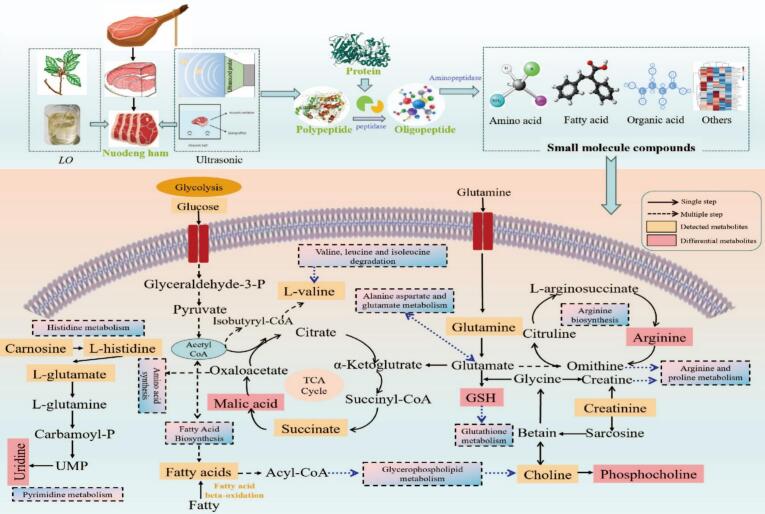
Analysis of the effect of ultrasound combined with *Litsea pungens Hemsl* essential oil compound coating on small molecule metabolic pathways network, yellow represents the metabolites identified in this study, and red represents the key metabolites identified. (For interpretation of the references to color in this figure legend, the reader is referred to the web version of this article.)
